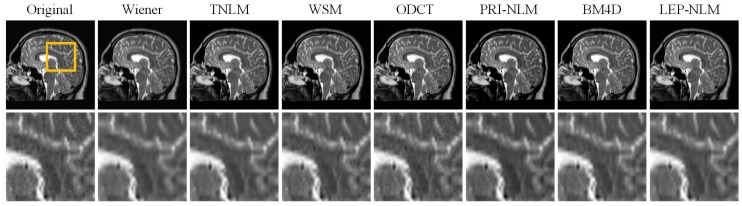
The restoration results of a real T2-weighted brain MR image by the various denoising filters. From top to bottom: the original MR images and the corresponding enlarged portions of ROIs marked with the yellow box in the original images.
